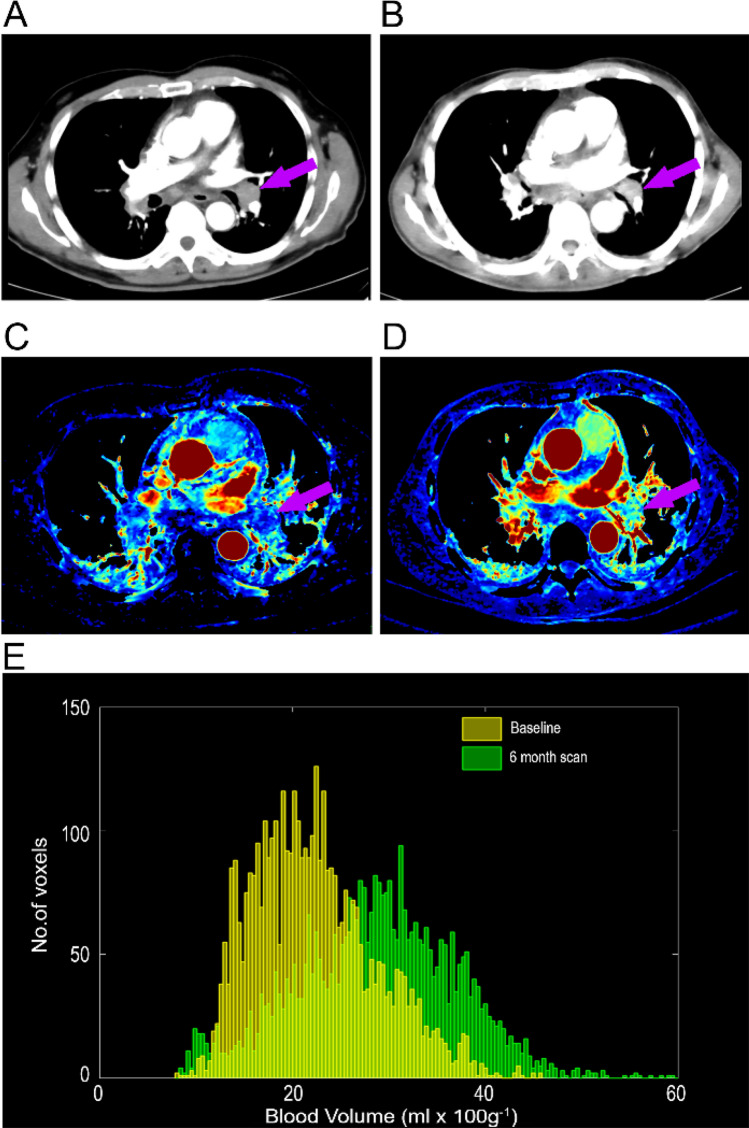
A patients with mRCC and a bronchopulmonary metastasis (purple arrow) on a routine CE-CT (A) at baseline and (B) the scan prior to progression, PDminus1; and DCE-CT (C) at baseline and (D) at PD. (E) The BV histograms of the metastasis depicts an increase in median BV values from baseline (21,57 mL × 100 g^−1^) to PDminus1 (28,85 mL × 100 g^−1^). *CE-CT* contrast enhanced Computed-Tomography, *DCE-CT* dynamic Contrast enhanced Computed-Tomography, *PDminus1* scan prior to progressive disease.
